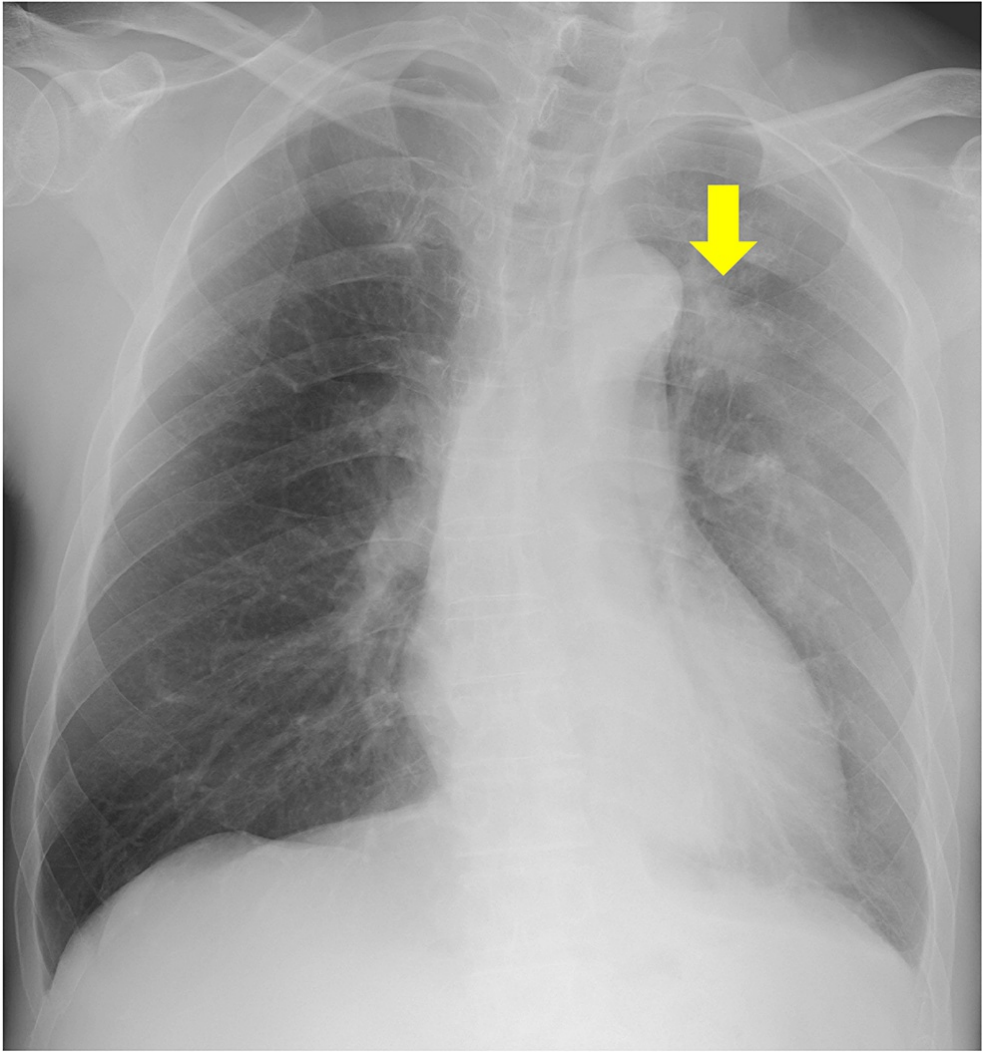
Chest radiography on admission shows a mass shadow in the upper left lung field (arrow).
